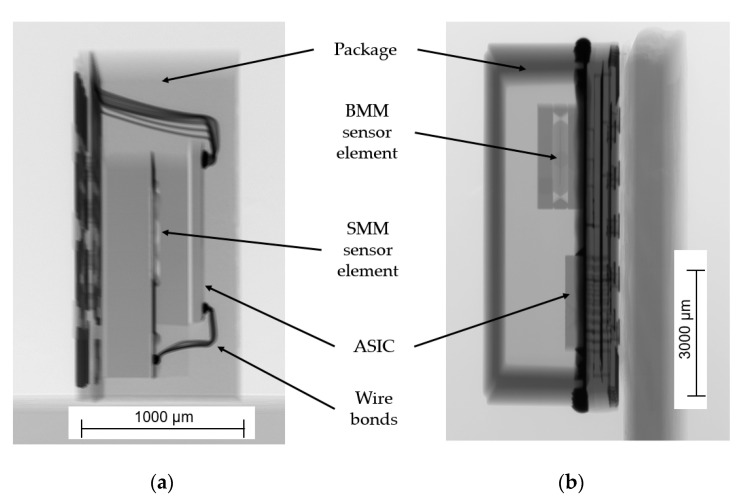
X-ray images of silicon MEMS acceleration sensors (not to scale). Images were made with a Nikon HMXST 160 computed tomography scan (CT): (a) Bosch BMA 280 with a three-axis SMM sensor element and a package size of 2 × 2 × 0.95 mm^3^; (b) Safran Colibrys SI1003.A with a one-axis BMM sensor element in an LCC20 package with a size of 9 × 9 × 3.3 mm^3^.
